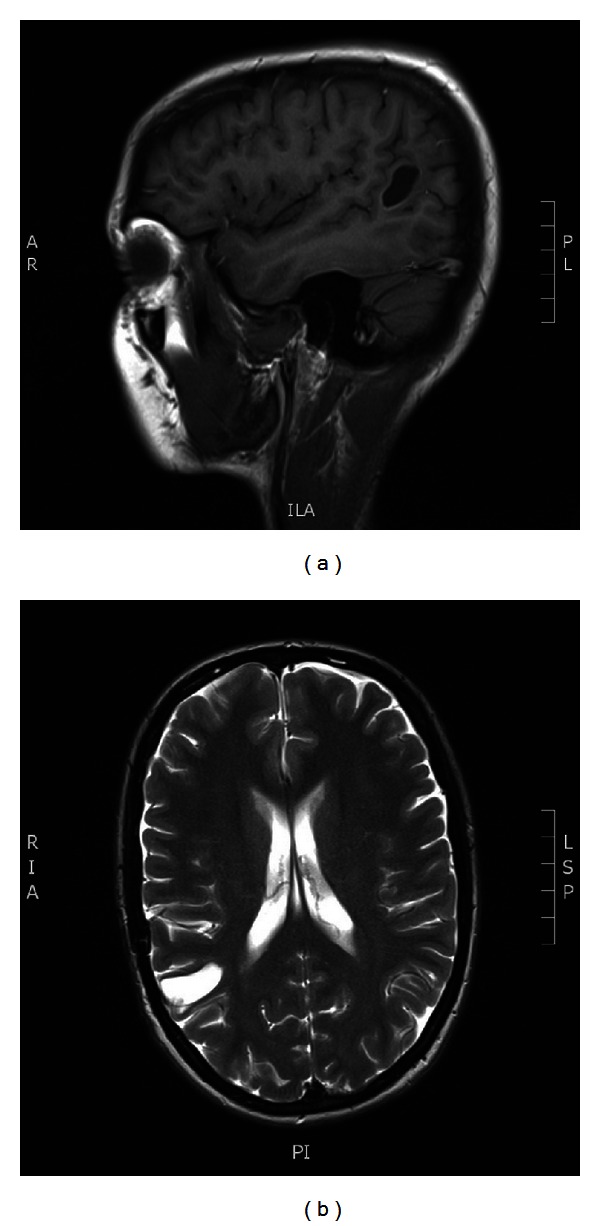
CT scan showing a hemangioblastoma in the right temporal lobe in the noncontrast and contrast series.
